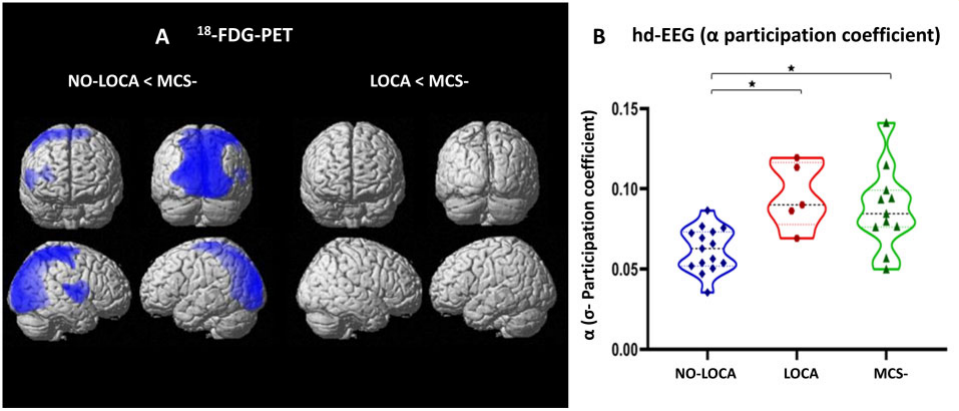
Results of ^18^FDG-PET and hd-EEG analyses comparing UWS LOCA and NO-LOCA patients with patients in MCS minus. (A) With ^18^FDG-PET, we observed more hypometabolism (blue spots) in NO-LOCA compared to MCS minus patients, whereas there was no significant difference between LOCA and MCS minus patients. (B) Hd-EEG analyses revealed a significant difference in alpha participation coefficient between NO-LOCA and MCS minus patients (*P* = 0.001), but not between LOCA and MCS minus patients.
